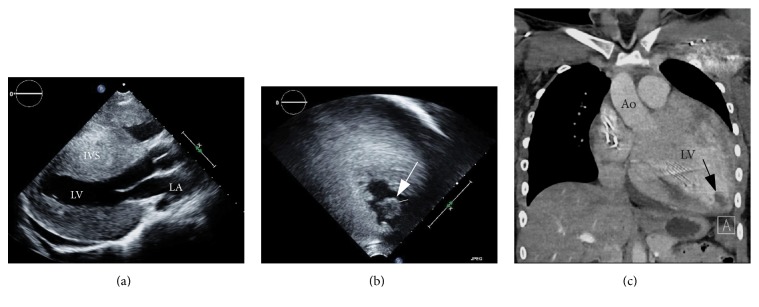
Images of hypertrophic cardiomyopathy (HCM) and a thrombus in the left ventricular (LV) apex. (a) Parasternal long axis view (a) showed severe concentric hypertrophy of interventricular septum (IVS) and LV free wall, but without an echogenic mass in the apex. (b) Off-axis apical four-chamber view identified a round echogenic mass in the LV apex measuring 1.3 × 1.4 cm (arrow). (c) A coronal reformat of postcontrast chest CT demonstrated cardiomegaly with an intracardiac thrombus at the LV apex measuring up to 1.8 cm (arrow). Ao: aorta, LA: left atrium, and LV: left ventricle.
